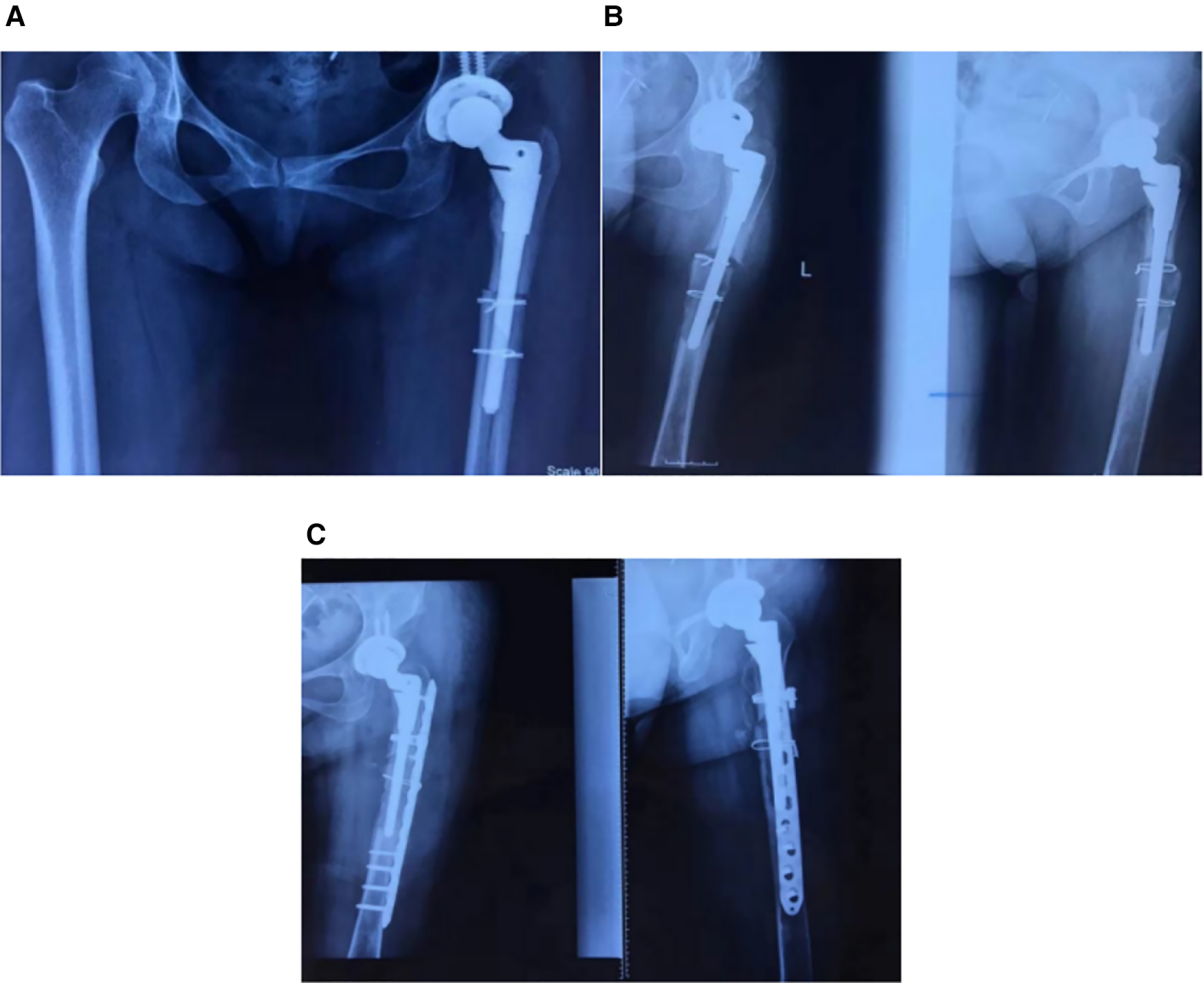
(A): anteroposterior radiographs of the pelvis after the patient's initial S-ROM prosthesis replacement are shown on the left, (B): radiographs of the hip joint with loose left hip prosthesis are shown on the right one year later. (C): Anterior and lateral femur radiation after revision of the femur.
